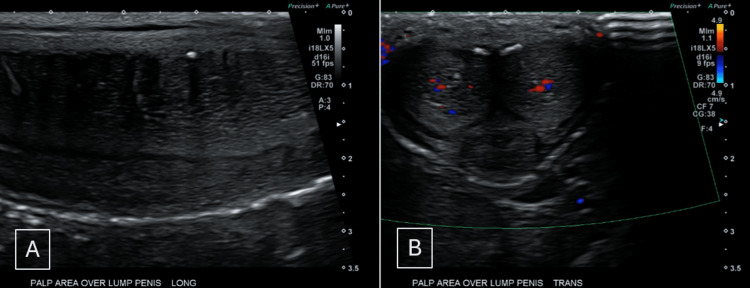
Penile ultrasound findings (A) Long-axis view demonstrating linear echogenic calcifications along the anterior medial corpus cavernosum corresponding to the palpable penile prominence. (B) Transverse view confirming focal tunical calcifications without a discrete mass, consistent with early Peyronie’s disease
